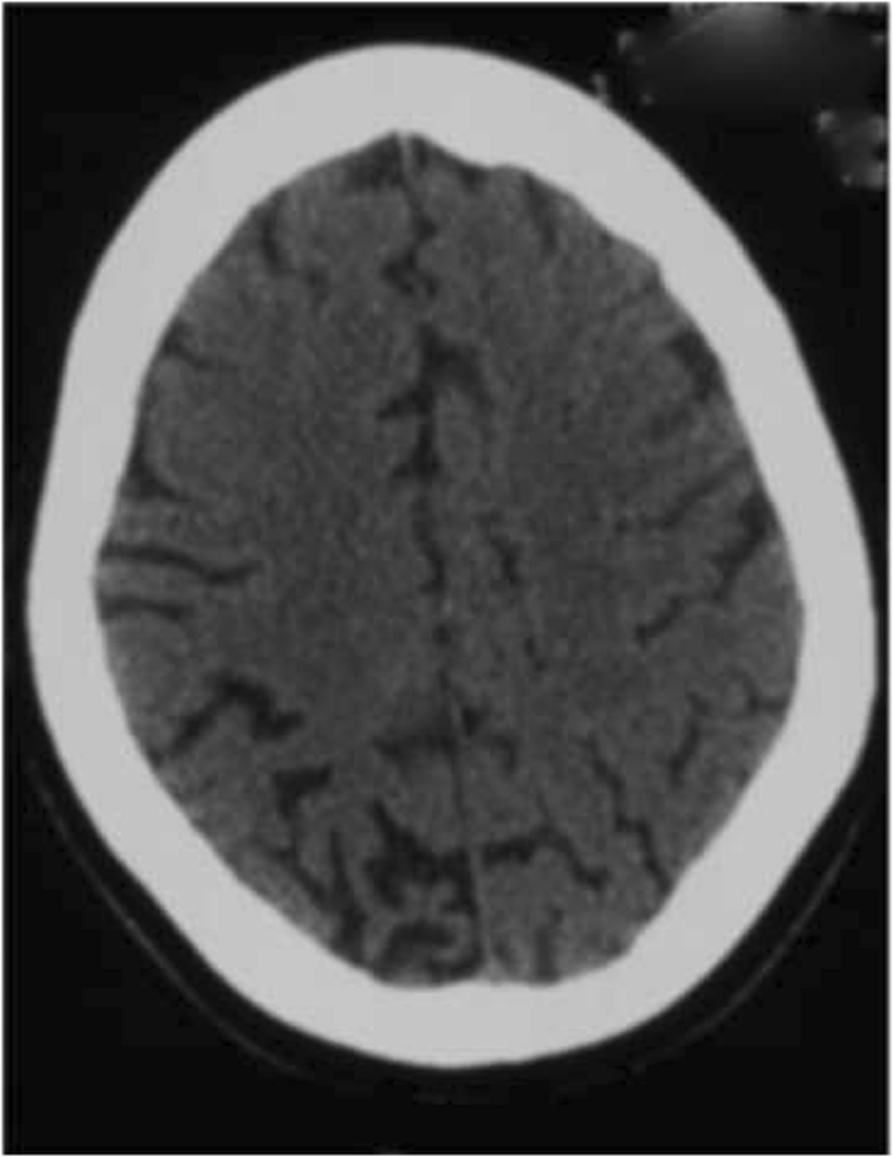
Brain CT-scan (March 2011): cerebral atrophy of the right posterior region.
